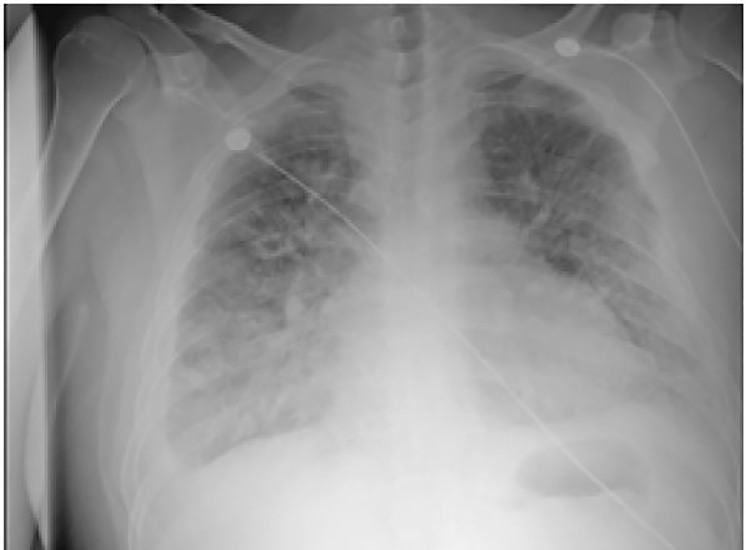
Initial anterior-posterior chest radiograph demonstrating diffusely hazy opacities in the lower lung zones and small bilateral pleural effusions, right larger than left.
